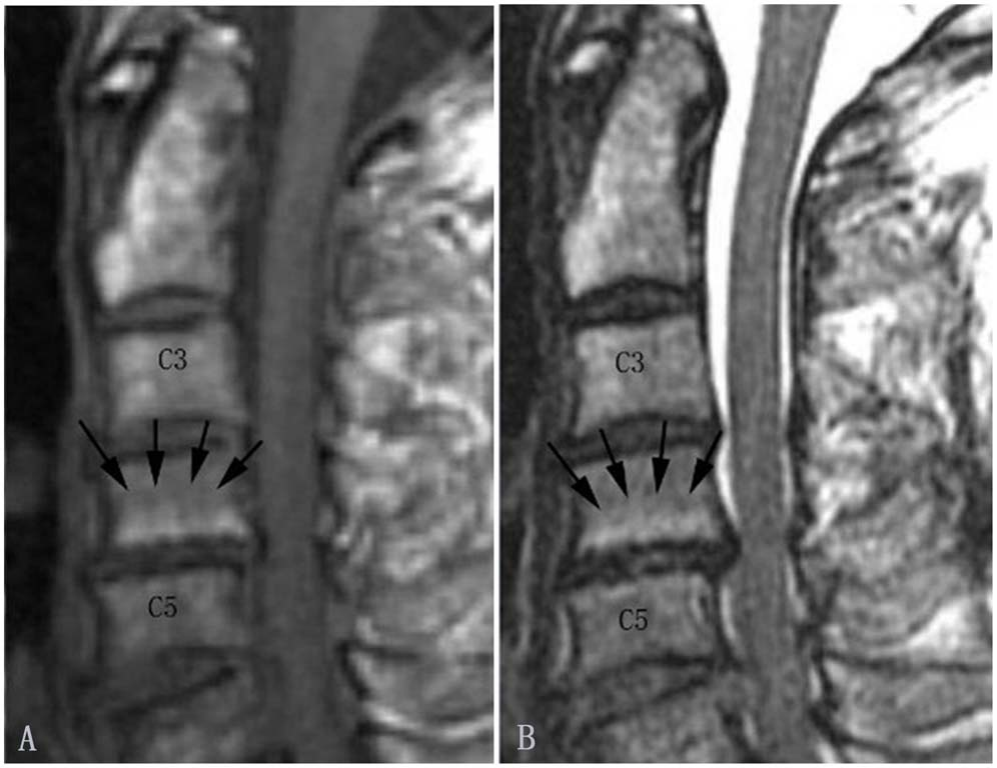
Modic type II changes in the cervical spine (indicated by arrows). Modic type II changes appear hyperintense on T1-weighted (A) and isointense or hyperintense on T2-weighted images (B).
